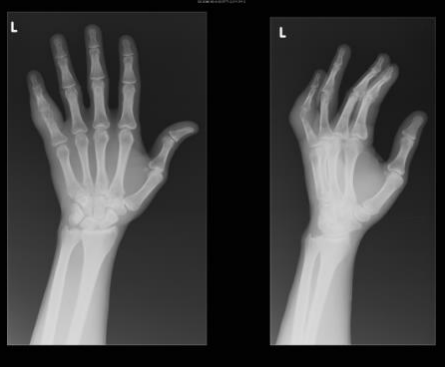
Plain radiograph of left hand demonstrated soft tissue swelling of the fifth digit, joint space narrowing with mottled lucency of the proximal phalanx, and cystic degenerative changes.
